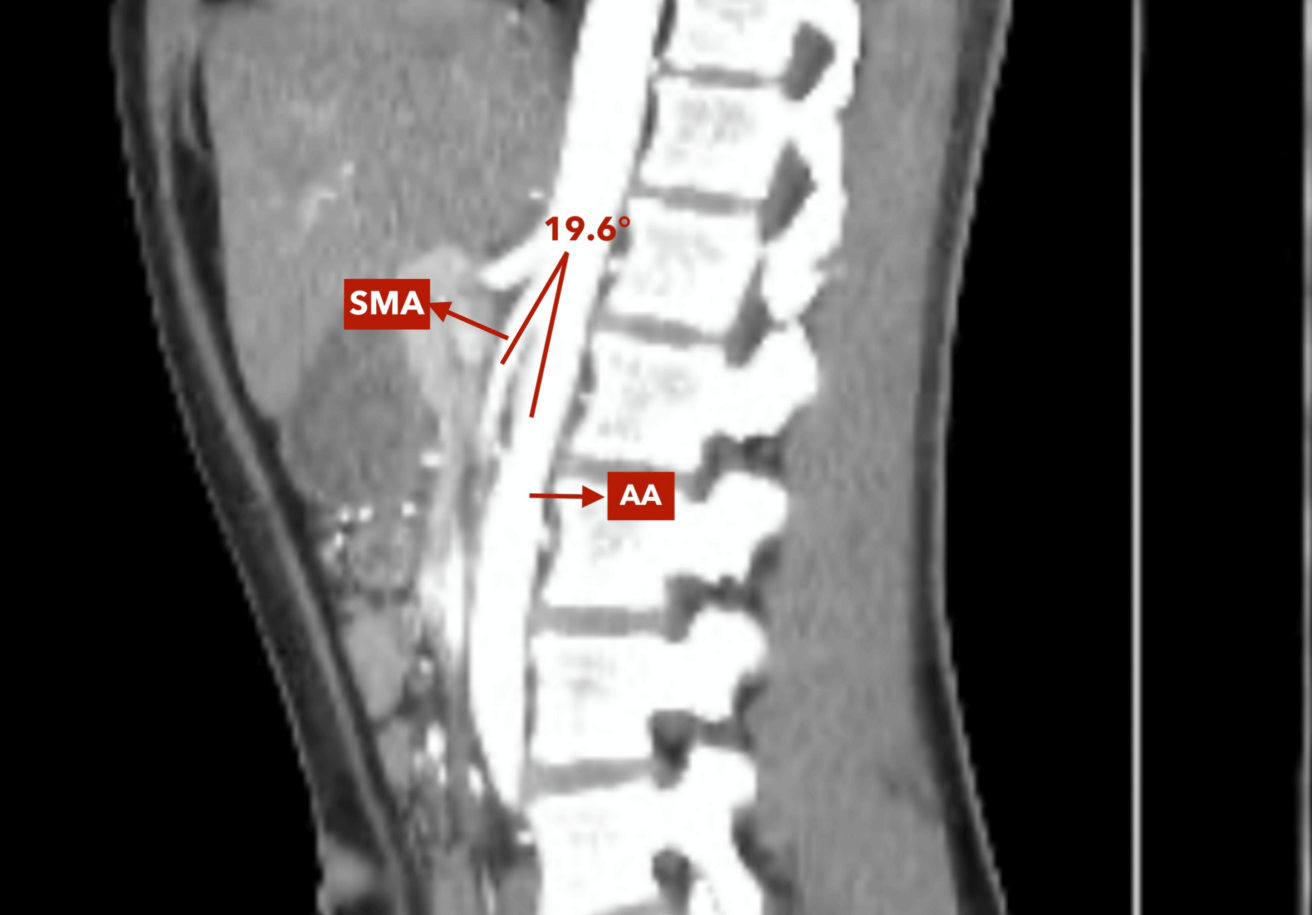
Zoomed-in sagittal reconstruction of contrast-enhanced abdominal CT scan (sagittal reconstruction, arterial phase) This image corresponds to a zoomed-in view of Figure 2, allowing closer visualization of the anatomical details and measurement of the aortomesenteric angle (19.6°) between the abdominal aorta (AA) and the superior mesenteric artery (SMA).
